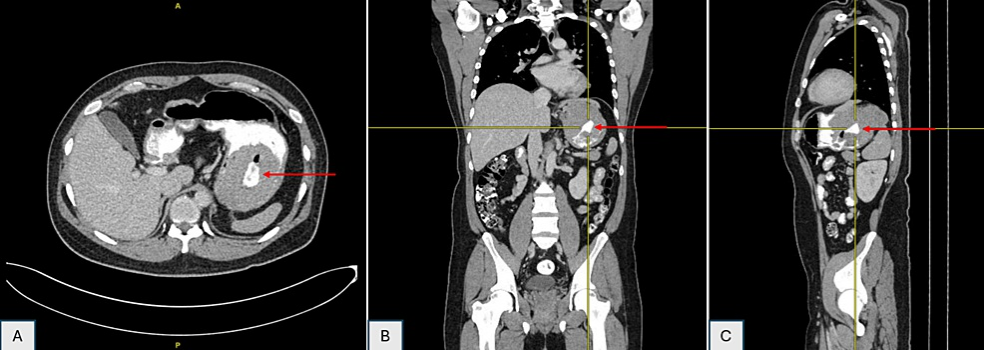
Exophytic component noted posteriorly on the CT thorax, abdomen, and pelvis A - axial view; B - coronal view, C - sagittal view
